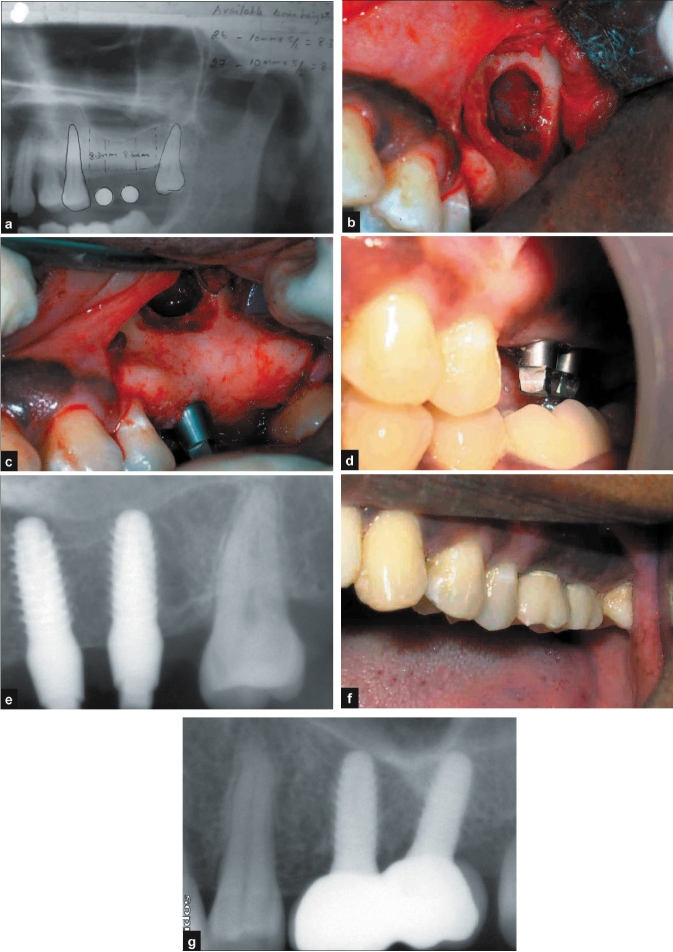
a) Pre-operative radiograph; b-e) operative photographs and radiograph; f, g) post-operative photograph and radiograph
